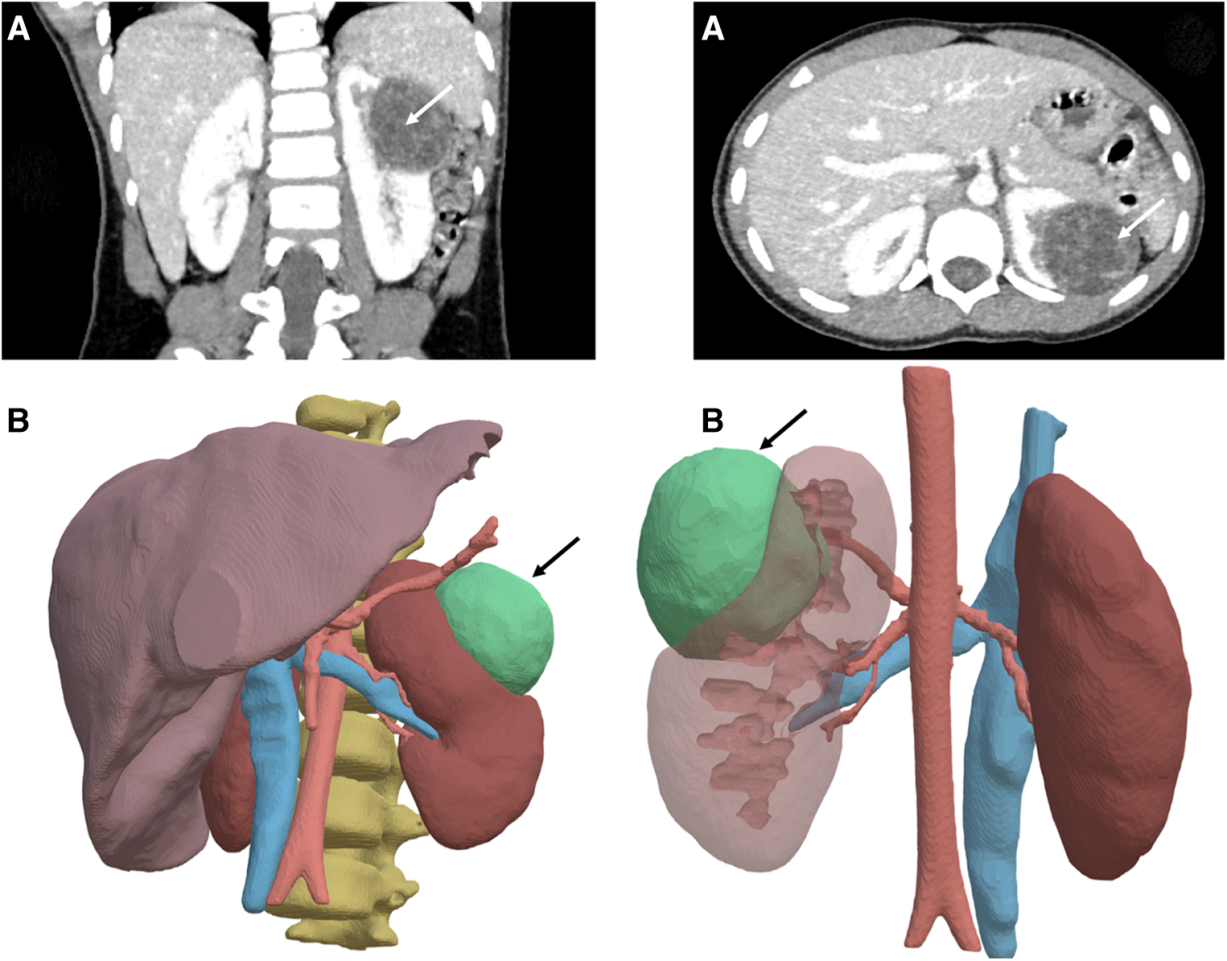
Left renal tumor and 3D modelling. (A) Computed tomography acquisition of a cystic nephroma in a 5-year-old boy with DICER1 syndrome. (B) Segmentation of the tumor (arrow) using semi-automatic methods in the IMAG2 laboratory-Paris-France.
